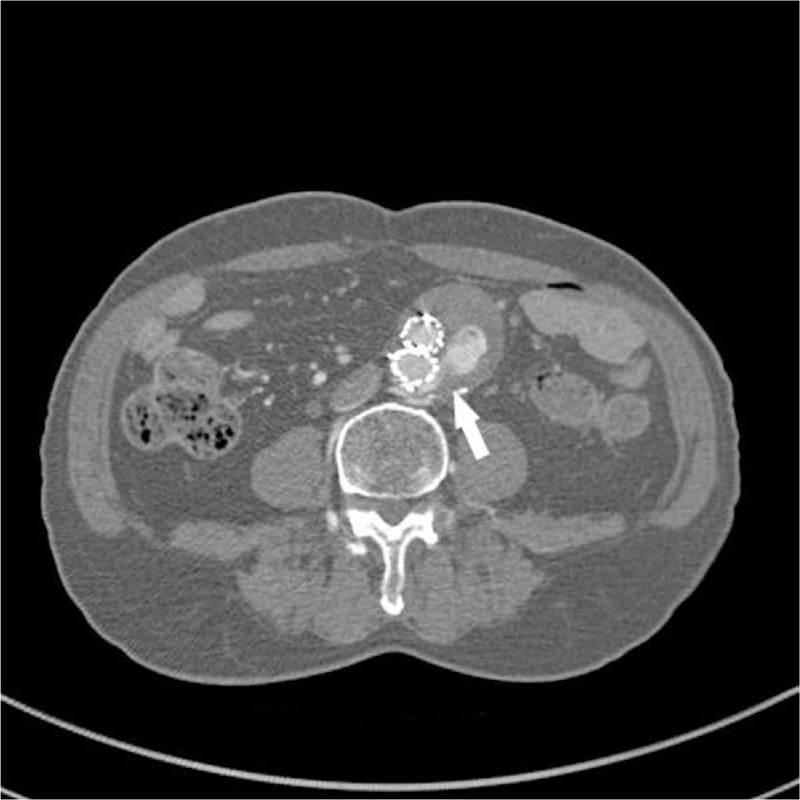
Axial view of two-year follow-up computed tomography angiography (CTA) taken 2 years after EVAR shows massive type II endoleak from lumbar arteries (arrow) with over 10 mm increase in sac diameter.
